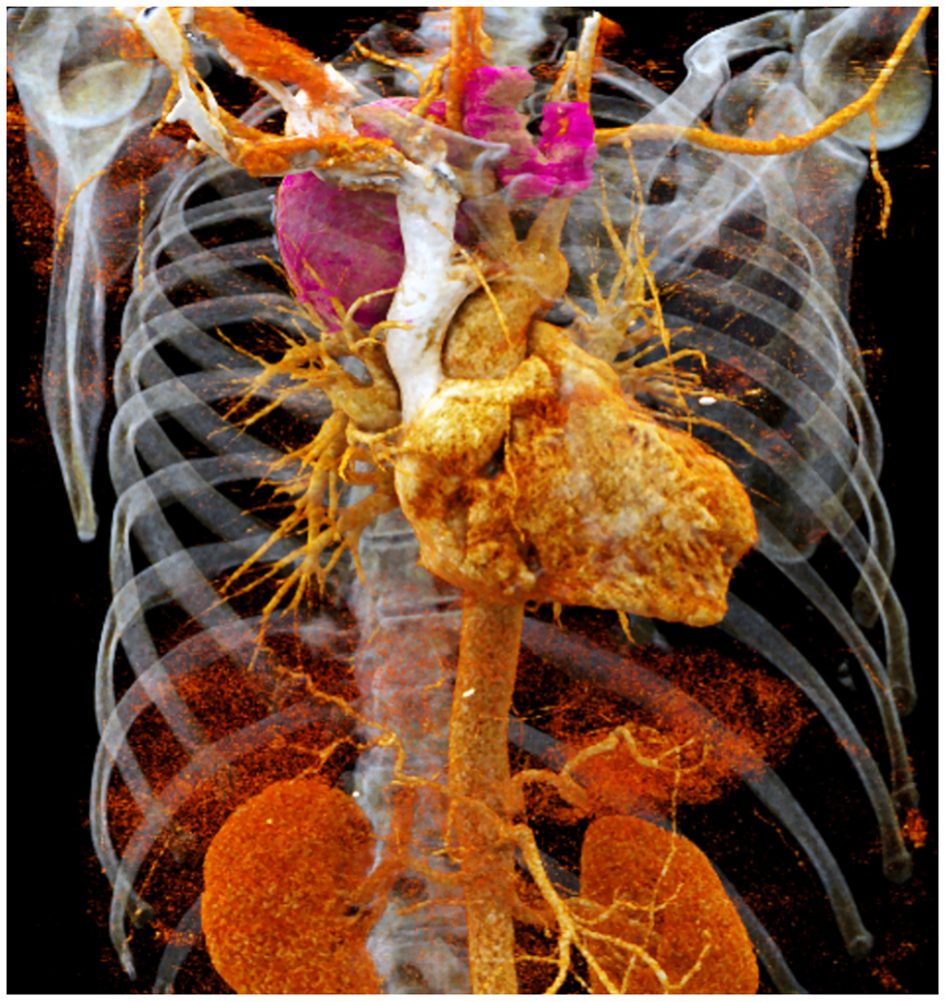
Three-dimensional computed tomography scan reconstruction showing a posterior mediastinal mass, most probably connected with the thyroid by a small tissue bridge and caudally in juxtaposition to the right upper lobe artery.
